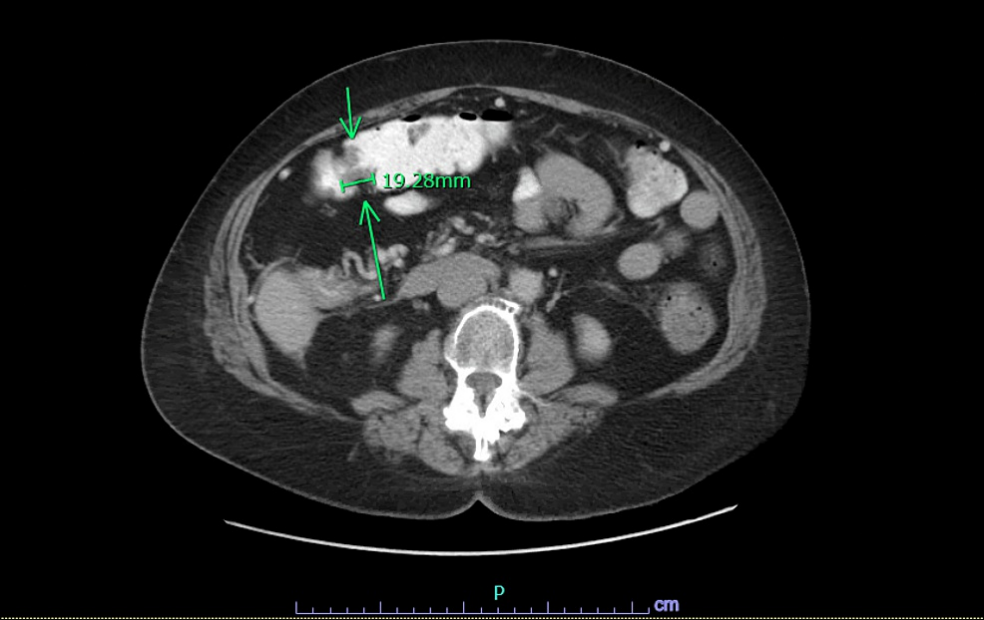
Repeat CT chest abdomen and pelvis shows a 1.9 cm concentric area of wall thickening involving the proximal transverse colon.
